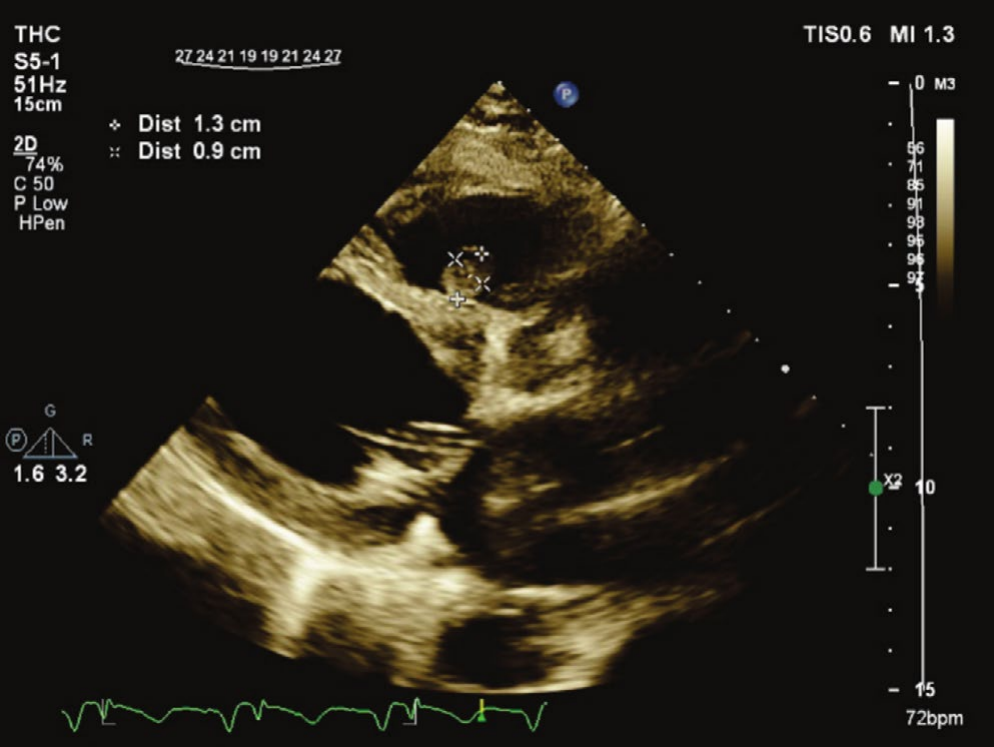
Transthoracic echocardiography in parasternal long axis, showing RVOT mass inserted close to pulmonary valve. RVOT, right ventricular outlet tract.
